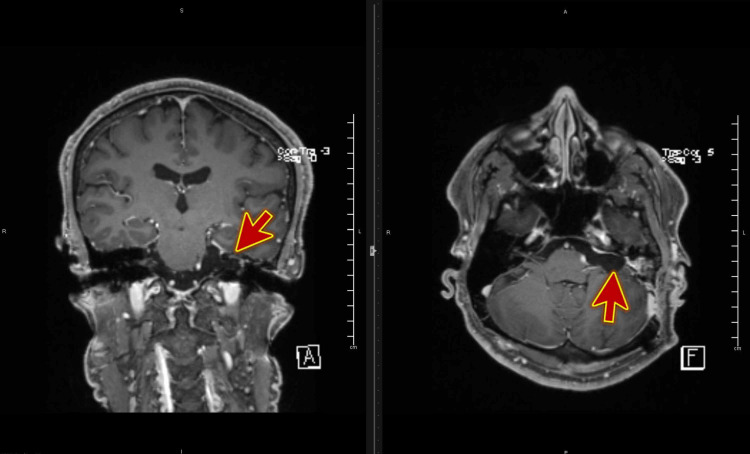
MRI scan of the head with contrast showing postoperative images of left acoustic neuroma.
